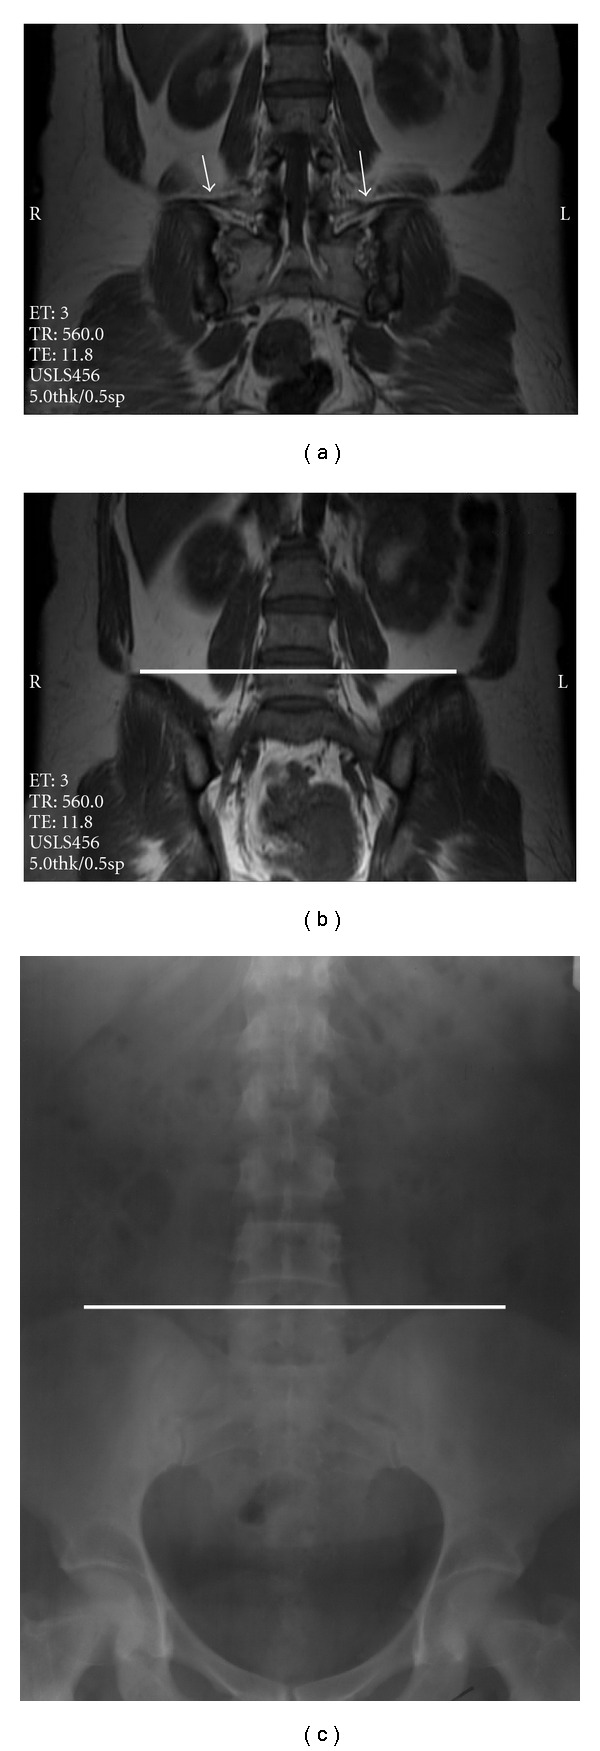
(a) Iliolumbar ligament (arrow) was used to identify L5 vertebra. (b) Coronal MRI showing Tuffier's line at the level of L5 upper segment. (c) Anteroposterior lumbosacral X-ray of the same patient which shows Tuffier's line at the same level.
